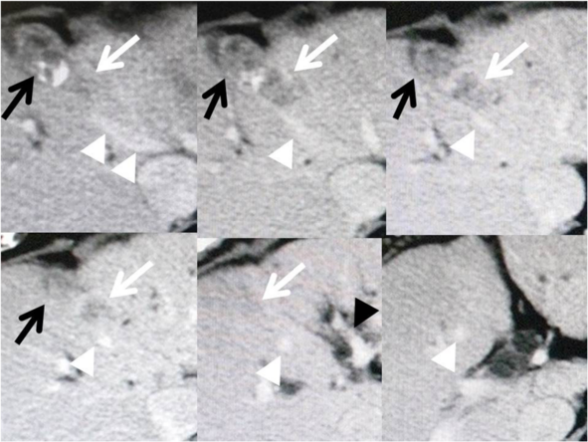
Computed tomography findings of the segment 4 tumor developing adjacent to the lesion previously treated with ablation. An irregularly shaped tumor (*white arrows*) was observed adjacent to the lesion previously treated with microwave tissue coagulation ablation (*black arrows*). An irregularly shaped tumor was broadly in contact with the middle hepatic vain (*white arrowheads*) and obviously separate from the umbilical portion of the portal vein (*black arrowhead*)
